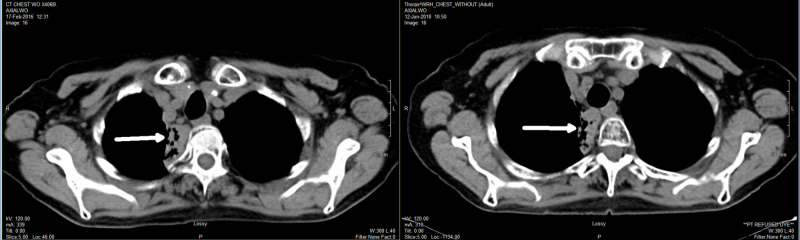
Follow-up CT showing CR with stable post-radiation changes in right lung in 2016 (left) and 2018 (right) CR, complete response
